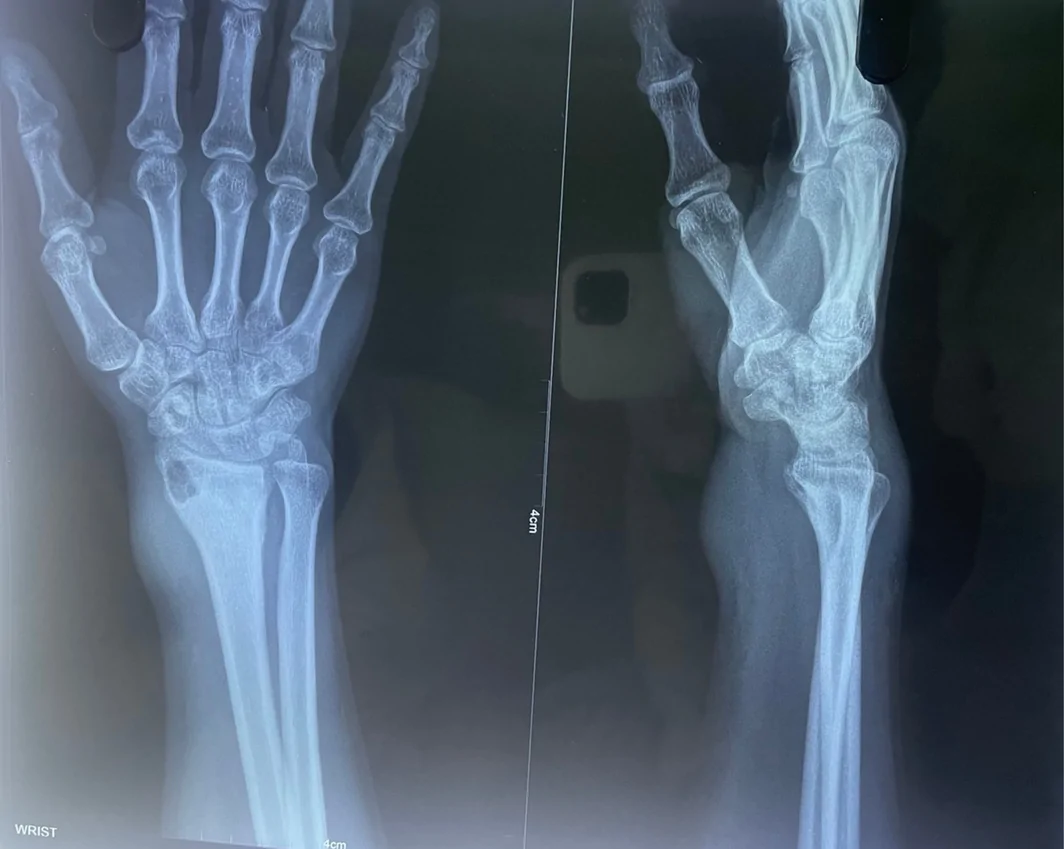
Plain radiograph of the right wrist demonstrating diffuse soft‐tissue swelling without obvious osseous destruction.
